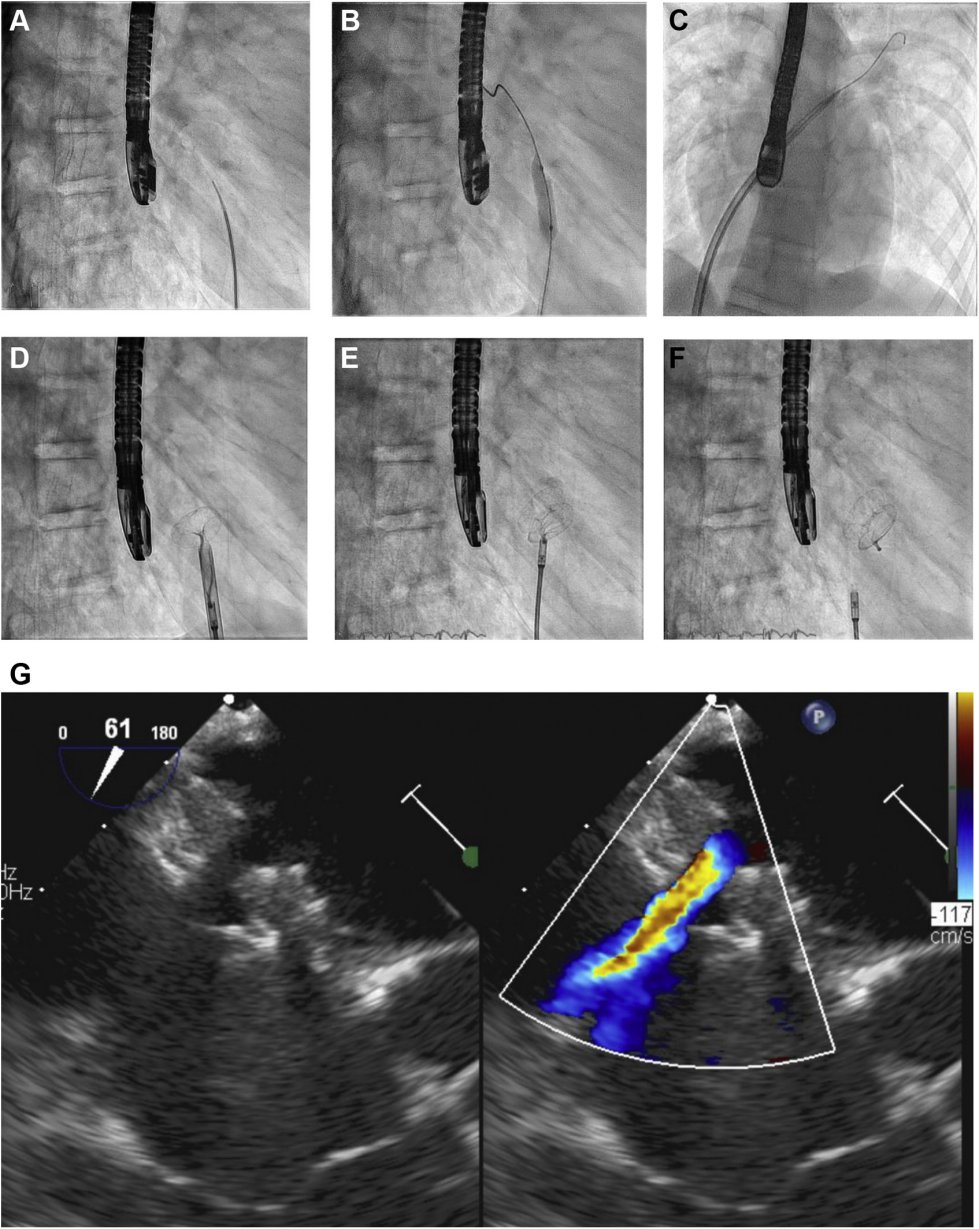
Interventional Percutaneous Implantation of the Atrial Flow Regulator-Device in a 13-Year-Old Girl With Restrictive Cardiomyopathy, Heart Failure With Preserved Ejection Fraction, and Mild Pulmonary Hypertension (A) Atrial transseptal puncture with Brockenbrough needle. (B) Balloon dilation of the atrial septum (Cordis Powerflex, 8 mm × 3 cm). (C) A guide wire has been positioned in the left upper pulmonary vein and a 12-F long delivery sheath is advanced across the atrial septum. (D) Deployment of the left atrial disc of the 8-mm atrial flow regulator (AFR) device. (E) The right atrial disc is deployed, and the AFR device is properly positioned. (F) After a pull maneuver, the AFR device has been released. (G) Transesophageal echocardiogram shows good position of the AFR device and restrictive atrial left-to-right shunt (6 mm).
